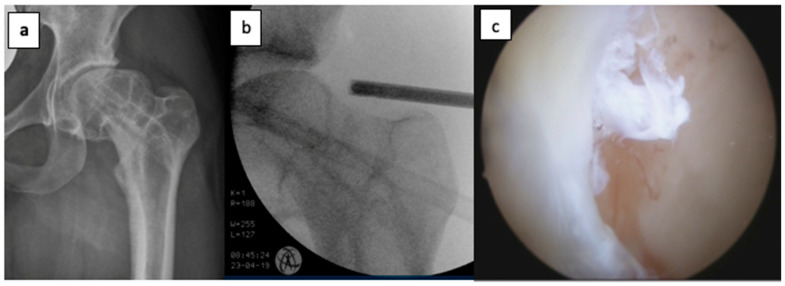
(a) Pre-operative X-ray of posttraumatic cam-impingement after slipped epiphysiolysis capitis femoris (SCFE); (b) intra-operative view of the same patient; (c) intra-operative labral tear.
